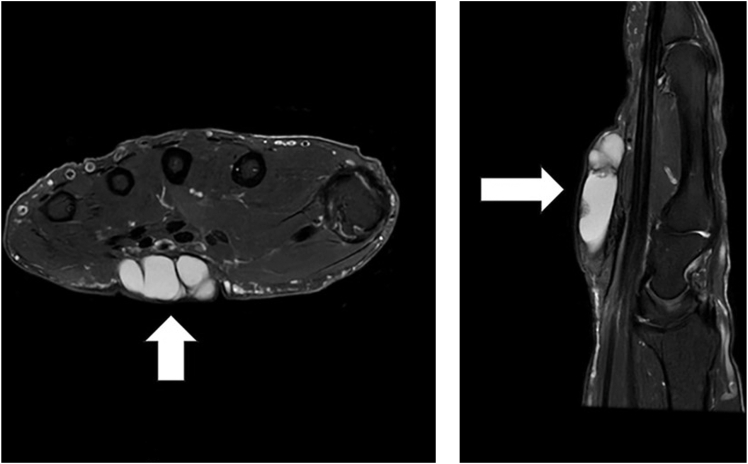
Preoperative MRI demonstrating a multiloculated cystic structure measuring 2.8 cm × 4.7 cm located between the skin and flexor tendon retinaculum (white arrows). Axial T2 image (left). Sagittal T2 image (right).
